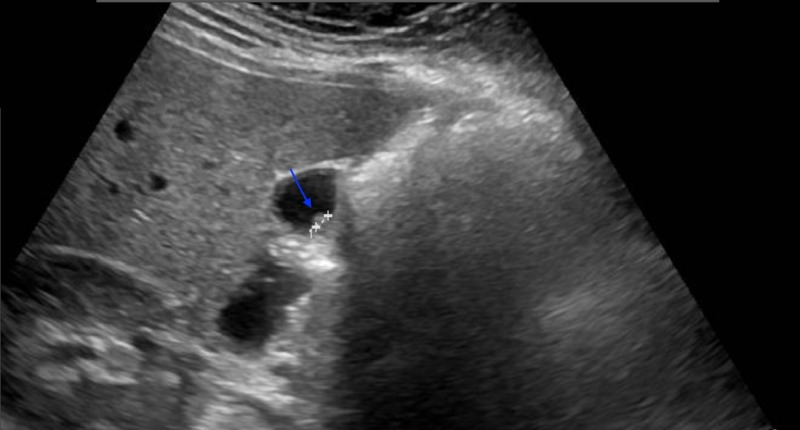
Ultrasonographic image of the polyp (blue arrow)
